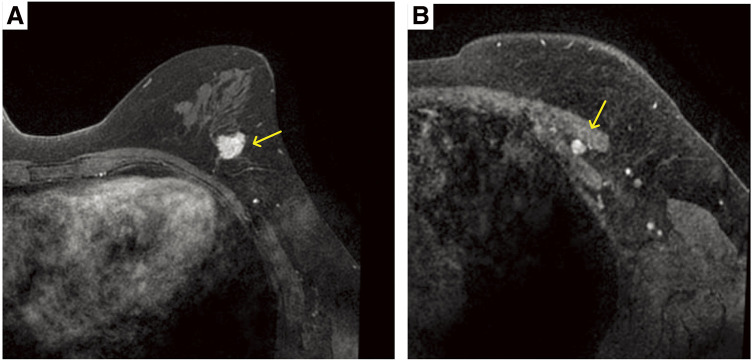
Contrast-enhanced breast MRI. (A) A mass in the left central (C) region showing rapid washout enhancement (arrow). (B) An enlarged, oval interpectoral lymph node with marked enhancement (arrow), suspicious for metastasis.
